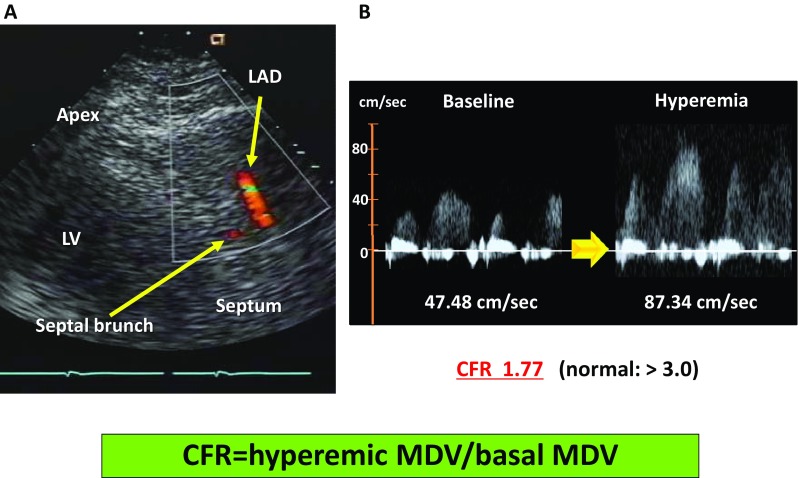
Measurement of CFR. a Visualization of the coronary artery using transthoracic echocardiography. b Coronary flow velocity at baseline and during hyperemia in patients with abnormal CFR. a Imaging of LAD using transthoracic echocardiography. First, the MDV at baseline is measured; thereafter, the MDV during a hyperemic condition induced by ATP administration (140 µg/kg/min IV) for 3–5 min b is measured. CFR is calculated as the ratio of hyperemic MDV to basal MDV. *CFR* coronary flow reserve, *LAD* left anterior descending artery, *MDV* mean diastolic velocity
